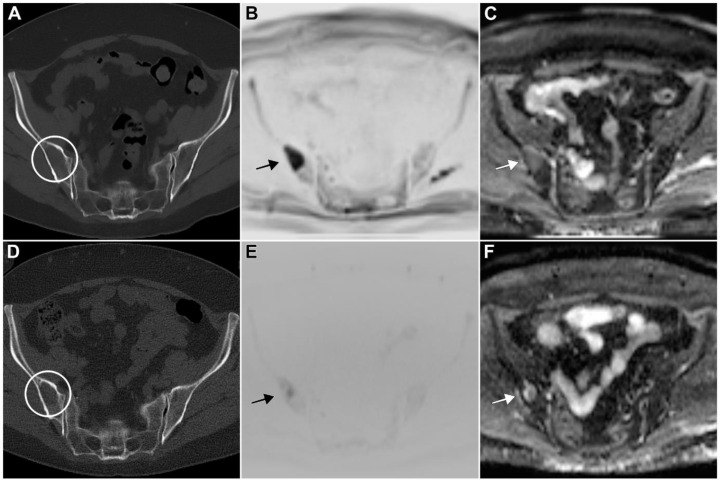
58-year-old woman with multiple myeloma (IgG kappa, R-ISS I) before (upper row) and after six cycles of E-KRd (lower row). Axial low-dose CT showed an osteolysis in the right iliac crest without size decrease or sclerosis after treatment ((A,D): circles). Signs of therapy response in diffusion-weighted MRI with residual disease ((B,E): low signal in inverted gray scale images with b-value of 2000 s/mm^2^; (C,F): incomplete ADC signal increase; arrows).
